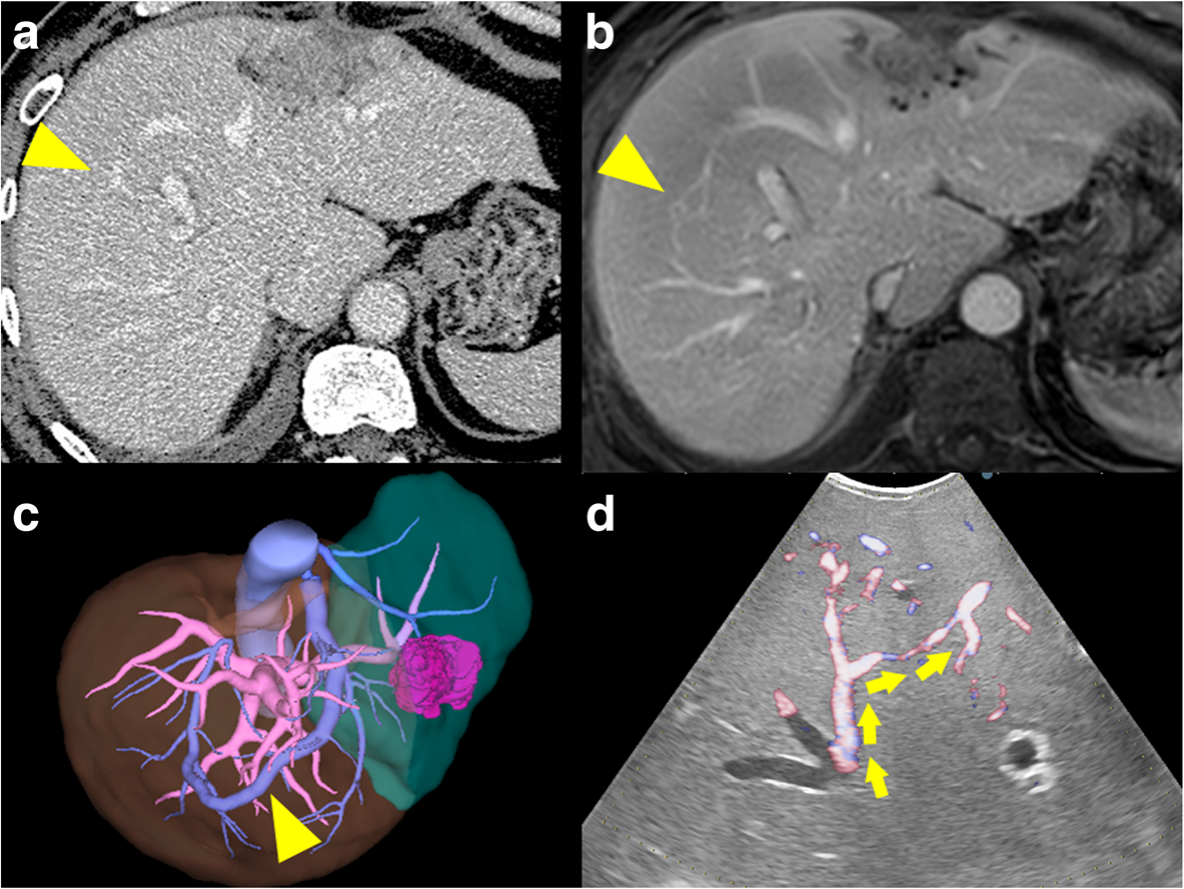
Development of intrahepatic venous collaterals (*yellow arrowhead*) between RHV and MHV after the first hepatectomy. a Contrast-enhanced computed tomography. b Contrast-enhanced magnetic resonance imaging. c Three-dimensional volumetric software. d Intraoperative Doppler ultrasonography. Intrahepatic venous collaterals derived from the RHV extended into the MHV (*yellow arrows*)
